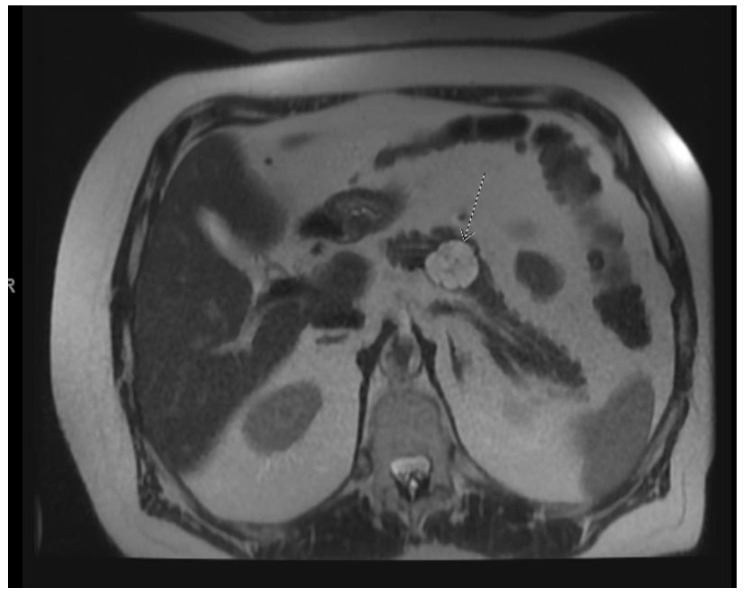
T2 weighted MRI of the abdomen demonstrating a serous cystic neoplasm with a central scar.
